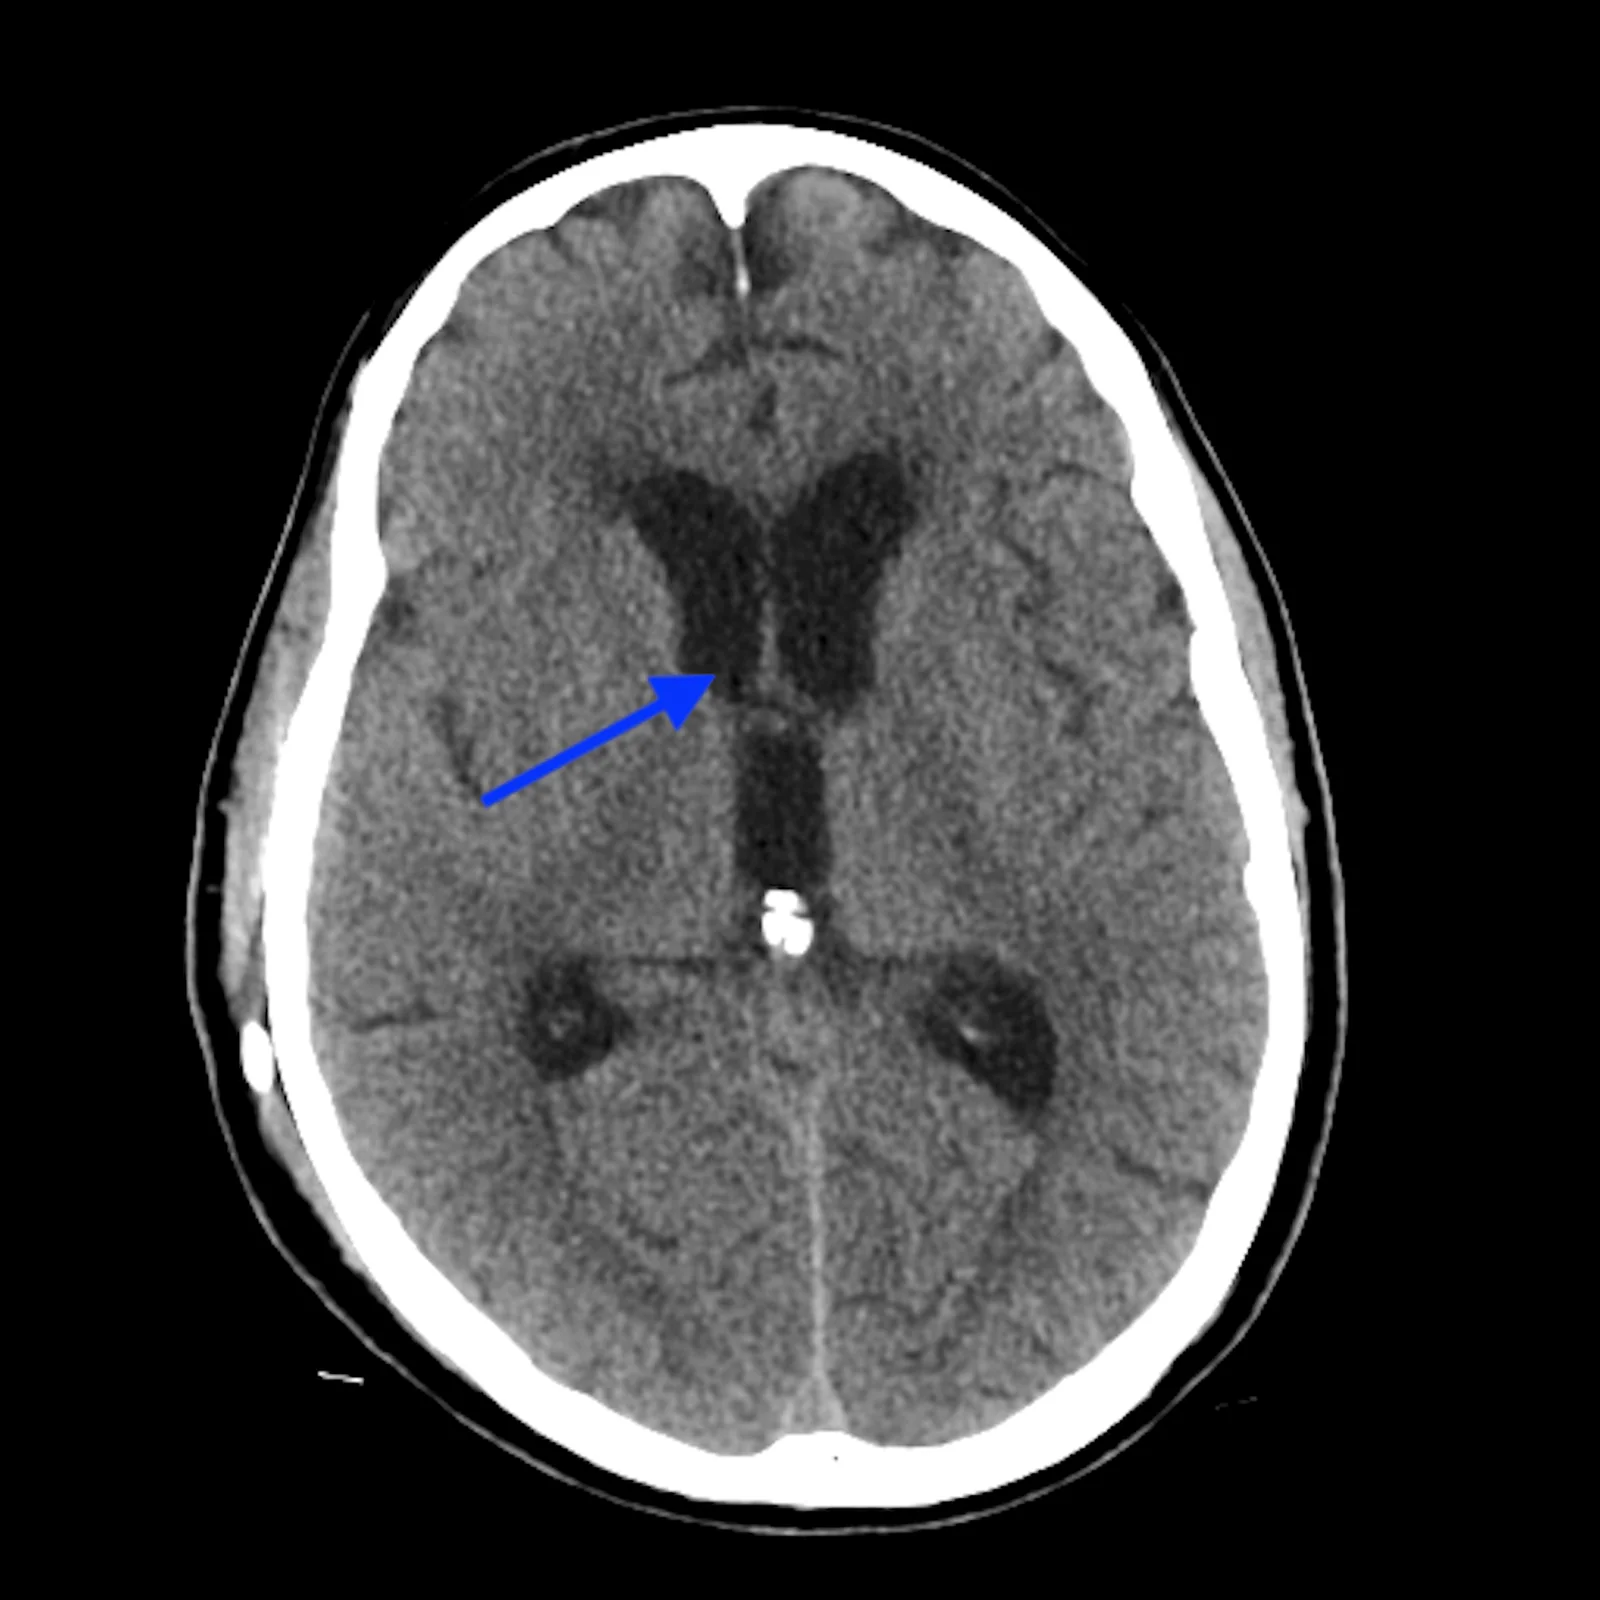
Axial non-contrast CT of the head following ventriculoperitoneal shunt insertion and before jugular venous compression, demonstrating persistent ventriculomegaly: third ventricular width 13 mm, temporal horns 6.2 mm (R) and 7.9 mm (L), and Evans ratio 0.30. Arrow indicates the right frontal horn. The ventricular catheter is not visualized on this section.
